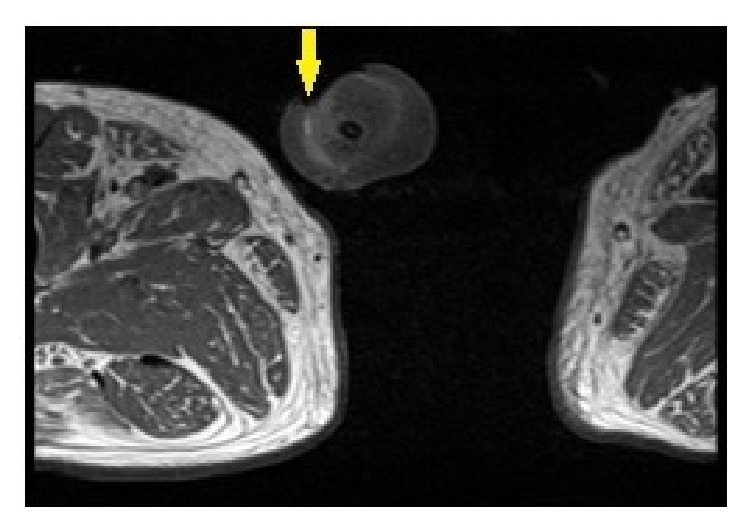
MRI pelvis showing T1 hyperintensity surrounding the glans penis, consistent with necrosis of the tip of the glans penis.
